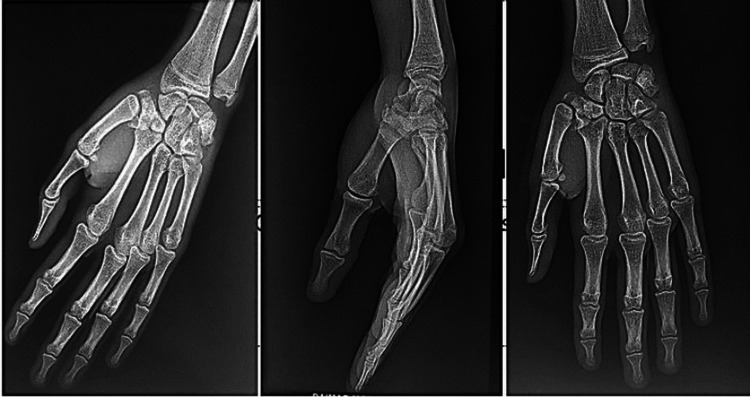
Standard X-ray of the patient showing a trapezium fracture associated with a Bennett fracture-dislocation.
